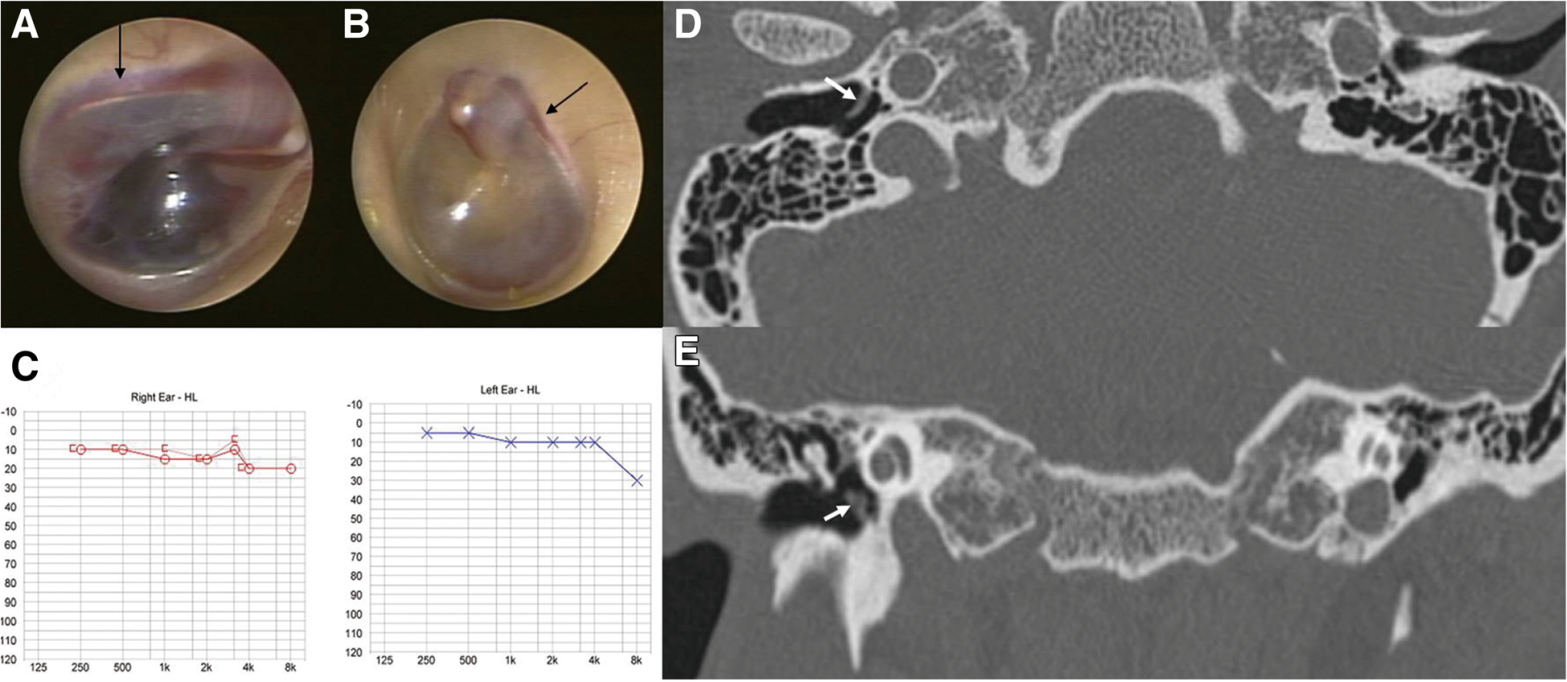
Otoendoscopic examination of patient 5 showed a small hemorrhage behind the intact tympanic membrane (TM) and along the line between the annular ligament and epidermis of the TM (black arrow) on the right side (a), and minor bleeding along the manubrium of the malleus and between the annular ligament and epidermis of the TM (black arrow) on the left side (b). Pure tone audiometry revealed no hearing loss on either side (c). Temporal bone computed tomography demonstrated mild thickening of the right TM on axial (d) and coronal views (e) (white arrows), suggesting iTM hemorrhage
